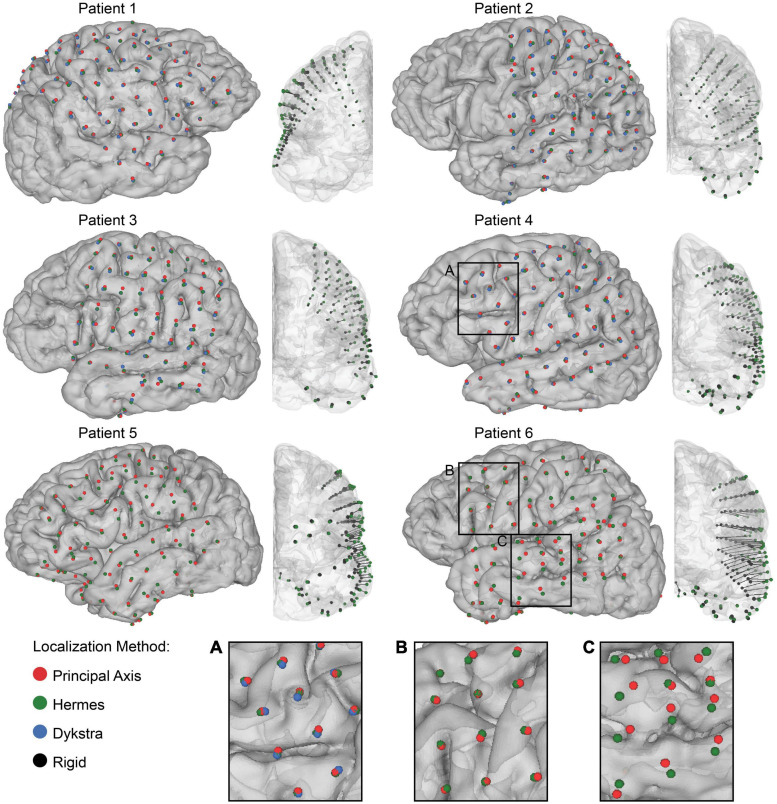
Electrode localization and quantification of brain shift. Final electrode localization for all projection methods for 0 mm CSF depth in each patient. The centroids indicate the final electrode localization on the patient-specific gray matter surface: principal axis (red), Hermes (green), and Dykstra (blue) (*left*) and the anterior view of the rigid electrode localization from the CT (black) with a vector to the projected Hermes localization on the gray matter surface (*right*). Both strip and grid ECoG electrodes are shown. Note that the Dykstra method did not converge for patients 5 and 6. Across projection methods, the centroid locations were similar for many of the patients (A), but varied in others by converging (B), and diverging (C) in separate brain regions.
